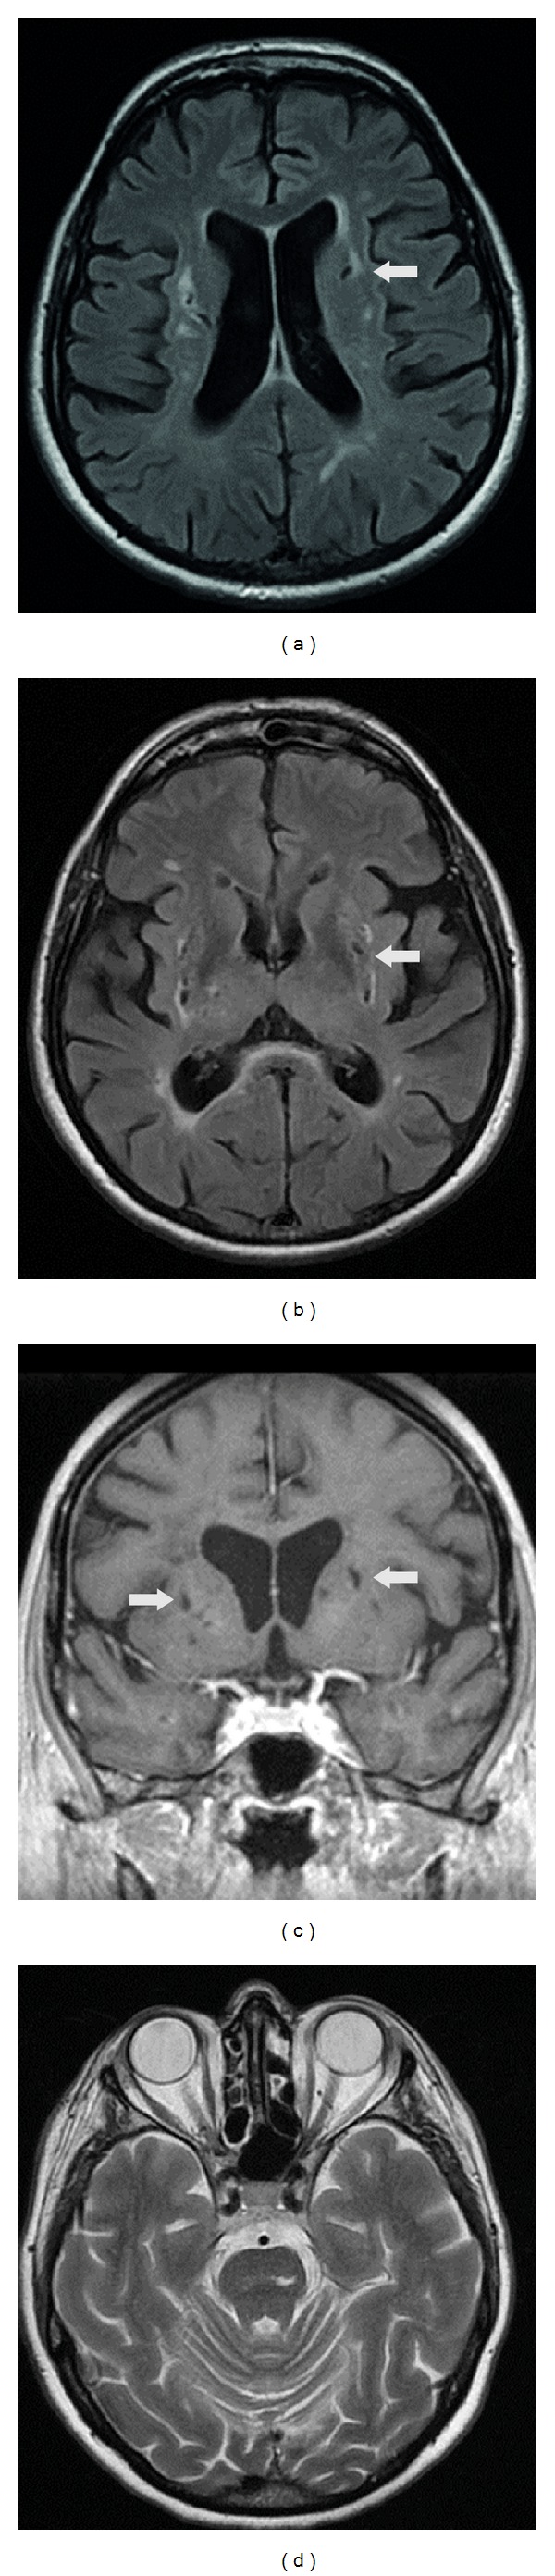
Cerebral MRI: lacunar state, with prominent lesions on the left caudate ((a and c) arrow), bilateral putamen ((b and c) arrow), and left pons (d).
